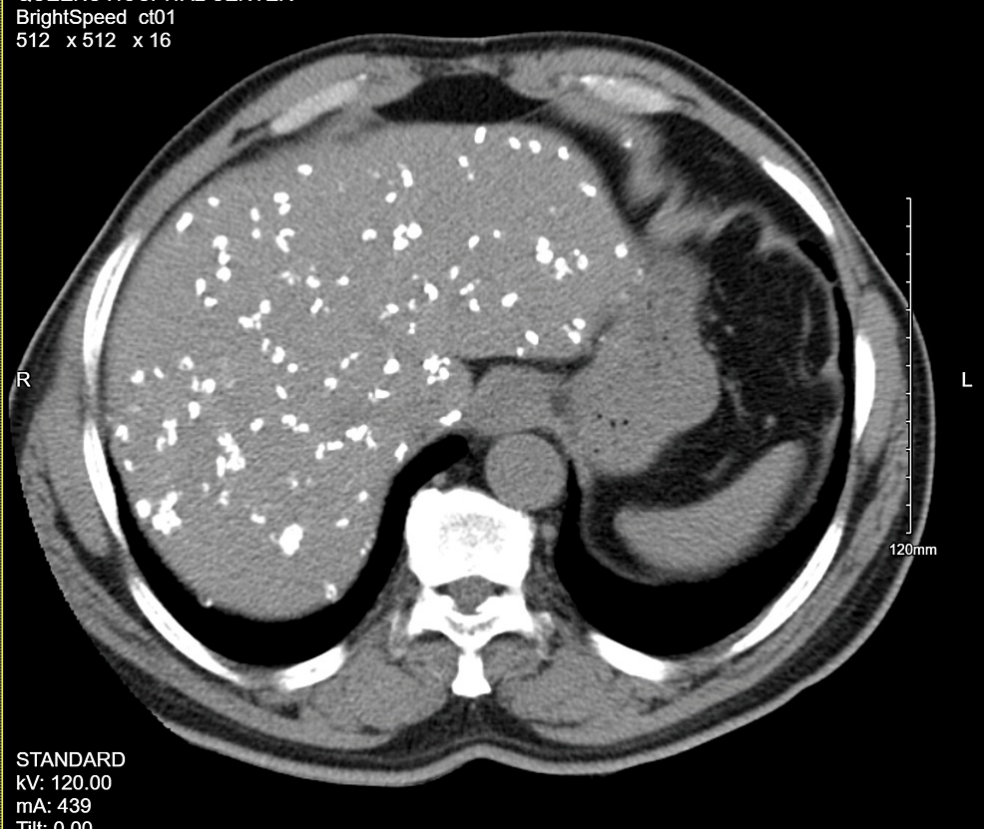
CT demonstrating radiodensities throughout the liver CT: Computed tomography
